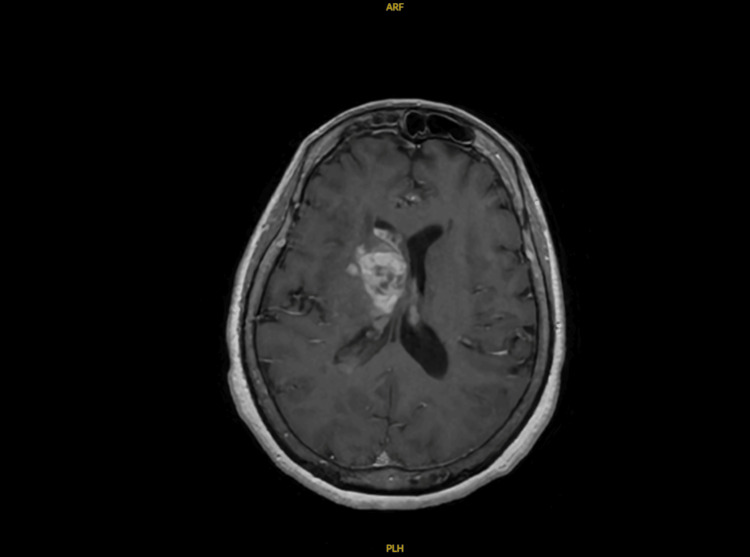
MRI T1 transverse stealth image showing a pattern of alternating hypointense-isointense and hyperintense rings in the right periventricular region, originally thought to be a cerebral abscess.
